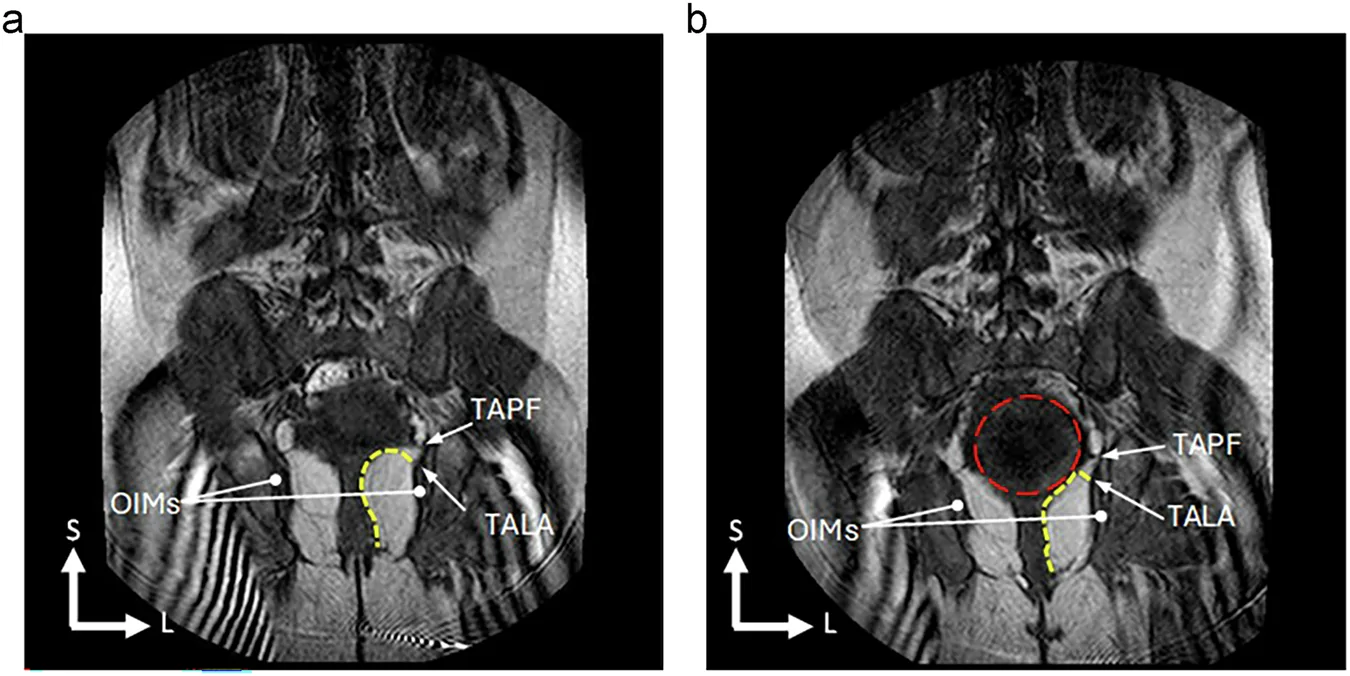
Coronal MRI highlighting the reversal of curvature of the iliococcygeal muscles (yellow dotted line) during labor (b). Left iliococcygeus convex side up before labor (a). Iliococcygeal concave side up after mobile fetal engagement marked with a red dotted line (b). Insertions of the TALA and the TAPF are highlighted (arrows). MRI, Magnetic resonance imaging; TALA, Tendinous arch of the levator ani; TAPF, Tendinous arch of the pelvic fascia
